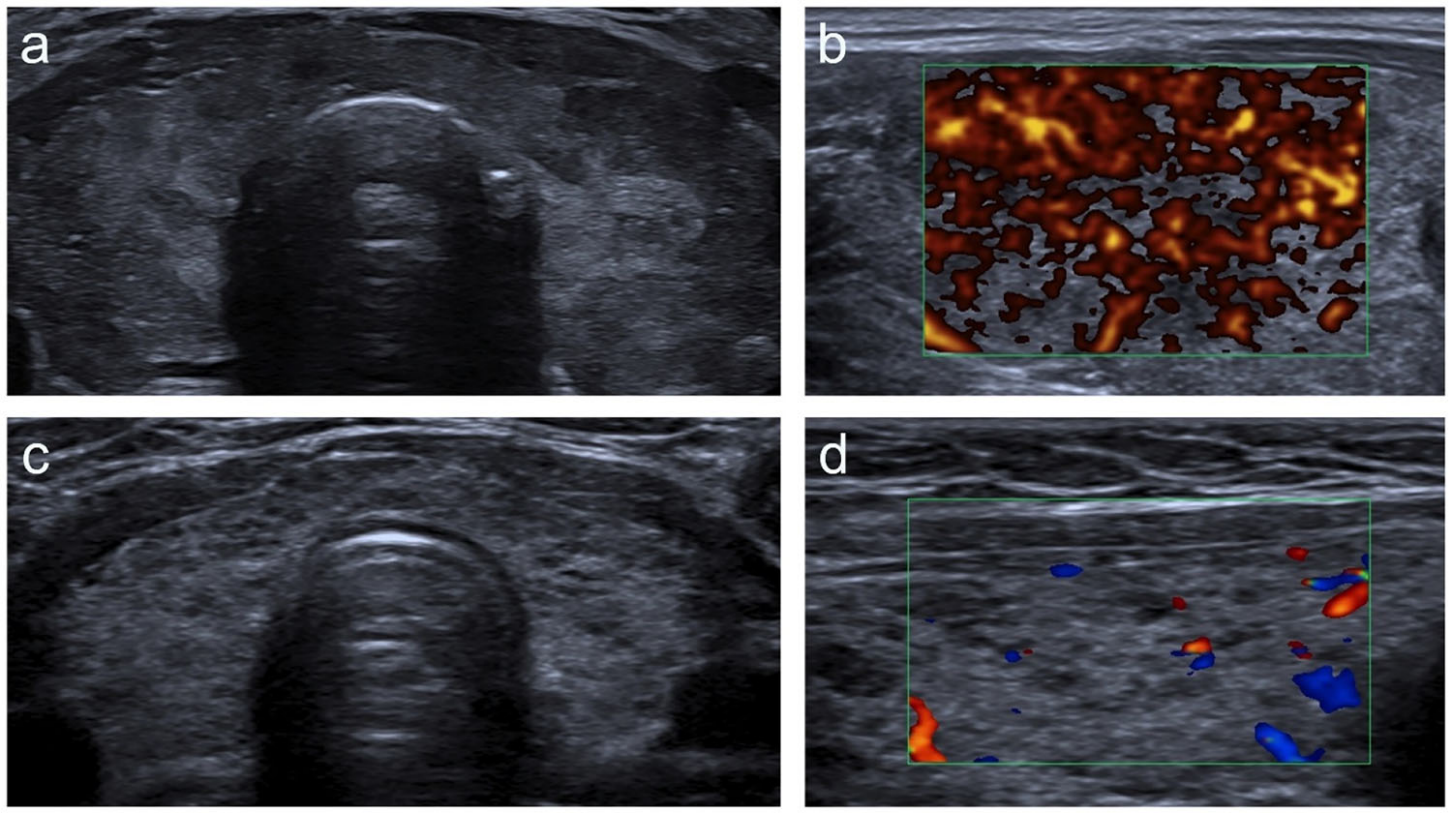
Sonographic features of diffuse thyroid disease in two different patients. a B-mode US in the axial plane demonstrates an enlarged thyroid gland with a coarse and hypoechoic echotexture, exhibiting a “thyroid inferno” pattern at Doppler interrogation (b) in a patient with Graves’ disease. c Axial B-mode ultrasound of a thyroid gland containing several hypoechoic foci scattered throughout its parenchyma due to lymphocytic infiltration, associated with reduced Doppler flow (d), consistent with Hashimoto’s thyroiditis
